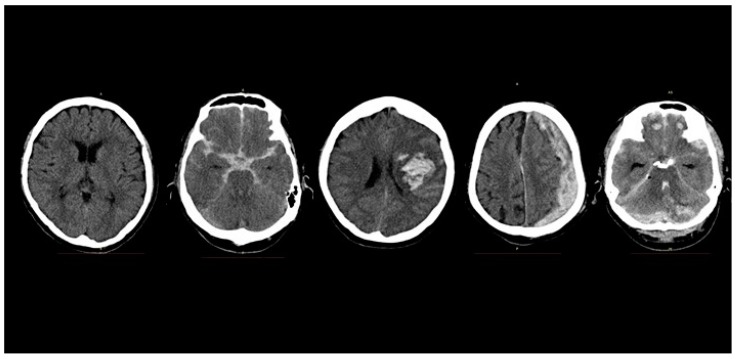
Computed tomography (CT) brain scans. From left, normal (N), subarachnoid hemorrhage (SAH), intraparenchymal hemorrhage (IPH), acute subdural hemorrhage (ASDH), brain polytrauma hemorrhage (BPH). Each image represents an individual image slice. One patient’s complete stack of CT images contained between 24 to 34 image slices in our dataset.
